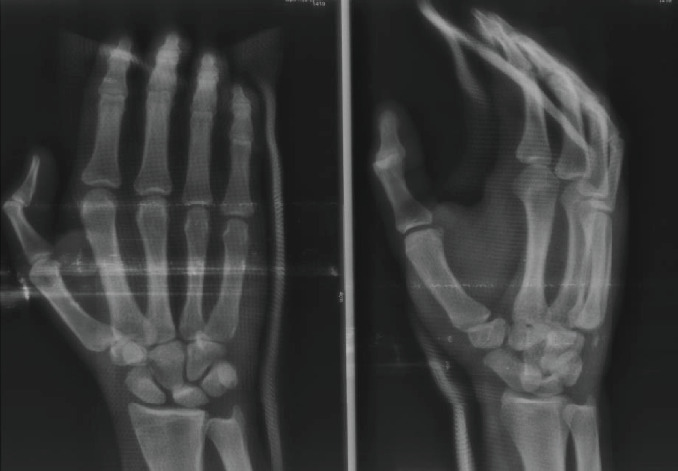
Anteroposterior and oblique radiographs of the right hand demonstrating complete dorsal dislocation of the three ulnar CMC joints.
